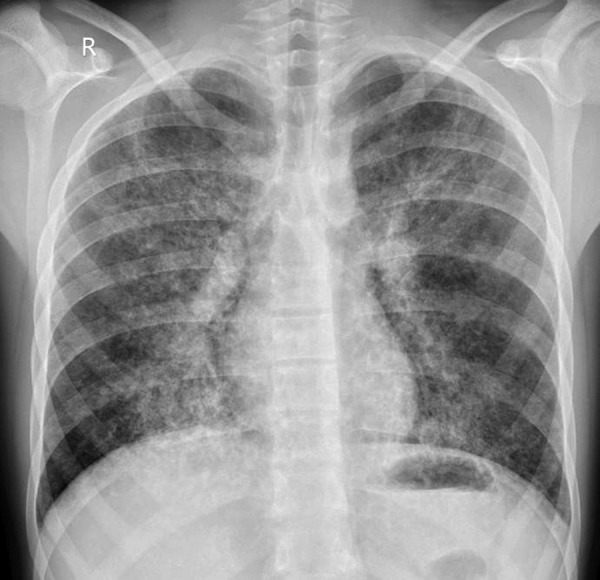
Chest radiograph. Fine bilateral interstitial infiltrates with interstitial edema.
